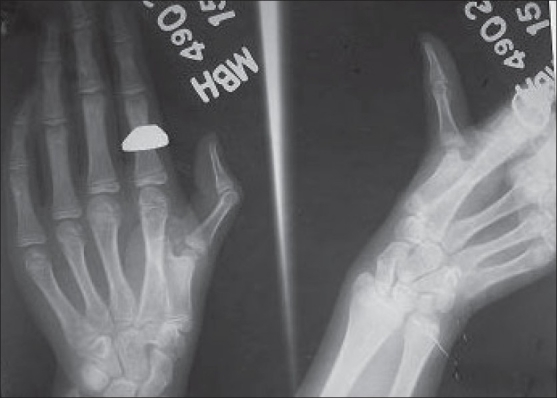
Anteroposterior and oblique radiograph of left hand including thumb showing bifid first metacarpal without its own epiphysis with hyperextention at metacarpophalangeal joint and flexion at interphalangeal joint
